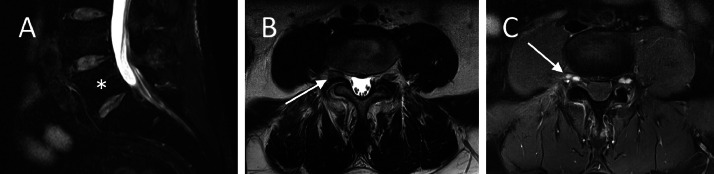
Sagittal T2-weighted image (A) showing a complete regression of the infiltration of L5 (star). Axial T2-weighted (B) and postcontrast T1-weighted fat-saturated sequence (C) image at the level of L5 showing complete regression of the infiltration of the right foramina and nerve roots (arrows).
